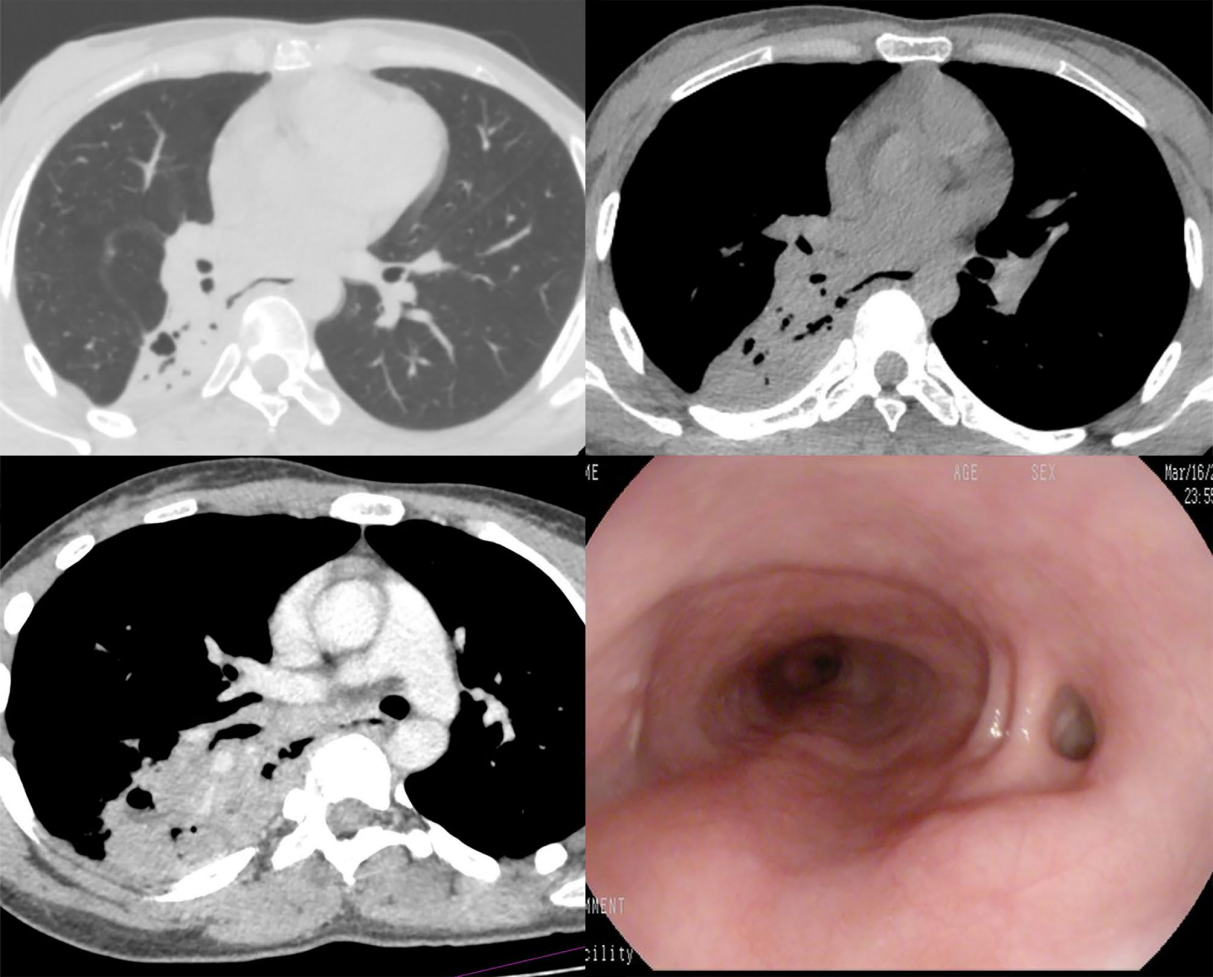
Columnar fistula; MSCT and contrast-enhanced CT images. Fistula observed by gastroscopy
